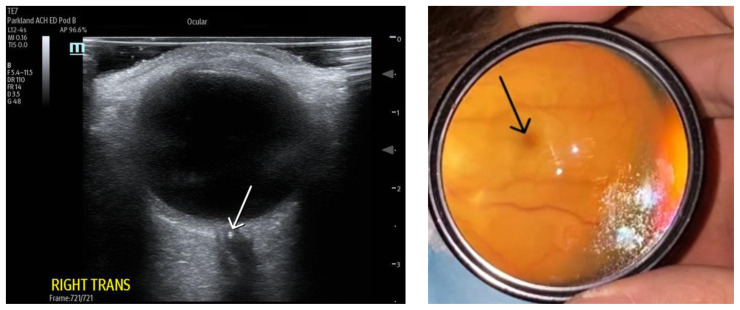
Ultrasound Video Link: https://youtu.be/miwLhjZ8WjA
